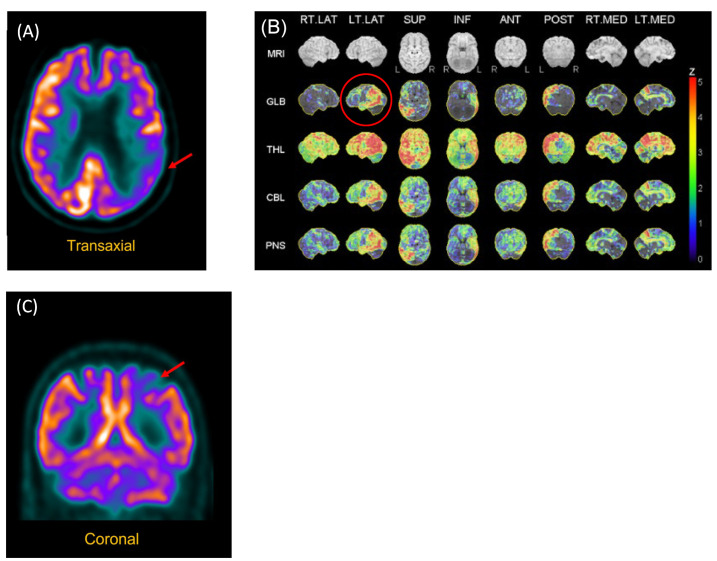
FDG-PET Scan of P2 and P3. (A) P2. Reconstructed slices showing hypometabolism of the left polymodal associative areas, especially the parieto-occipital regions, as well as asymmetry (left > right) of the primary visual areas. (B) P2. Statistical analysis of metabolism distribution as compared to marched normal database showing areas of hypometabolism in the left hemisphere, including in the left posterior temporal region (image circled in red). (C) P3. Hypometabolism of the left posterior parietal region.
